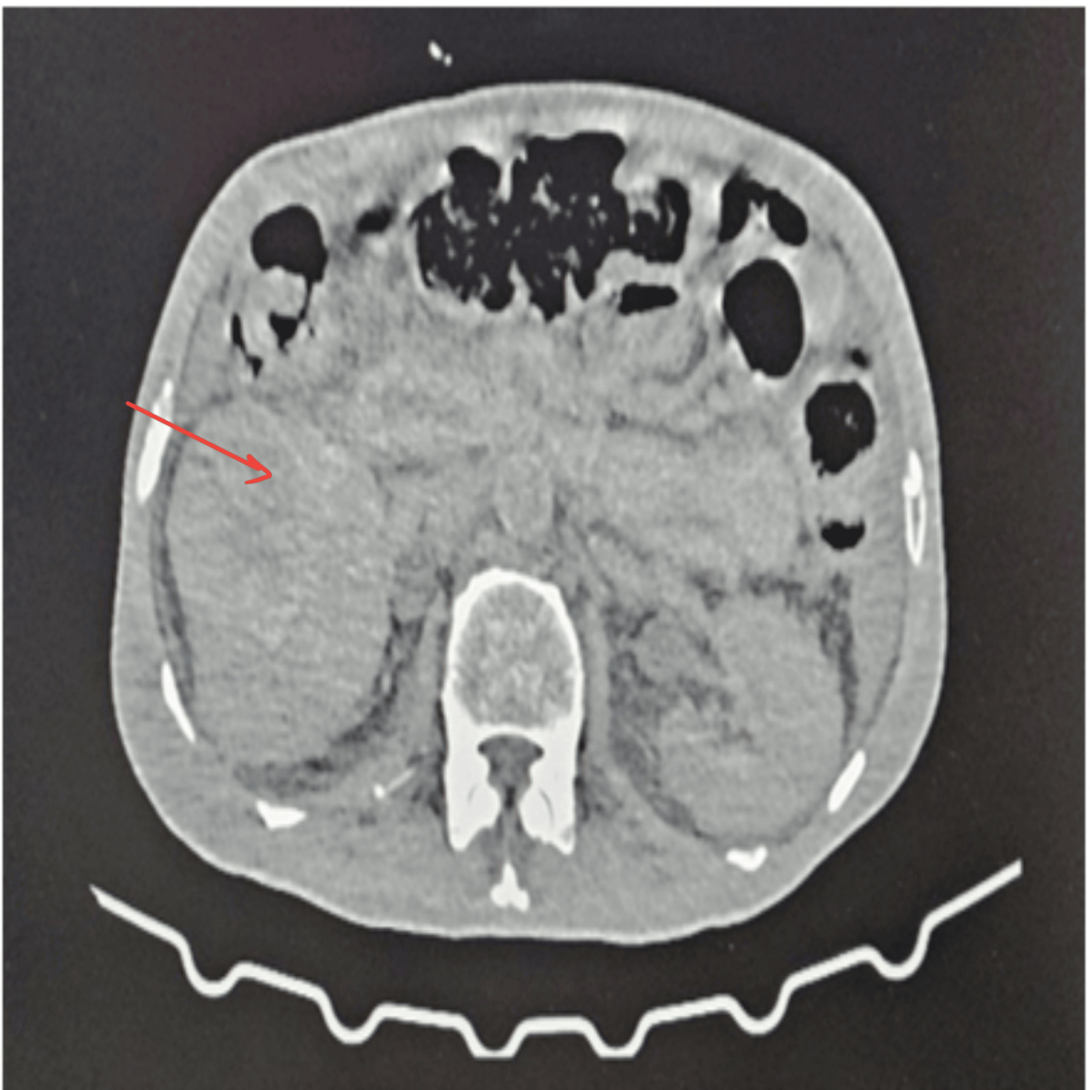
CT Abdomen showing enlarged right kidney measuring 14.9x6.7 cm (red arrow), multiple thick-walled hypodense collections in mid and lower poles of the right kidney (the largest measuring 7.3x4.1 cm) with minimal perinephric stranding. Right mild hydronephrosis is noted. The left kidney measures 11.4x4.6 cm with minimal perinephric fat stranding, which is suggestive of infective aetiology.
